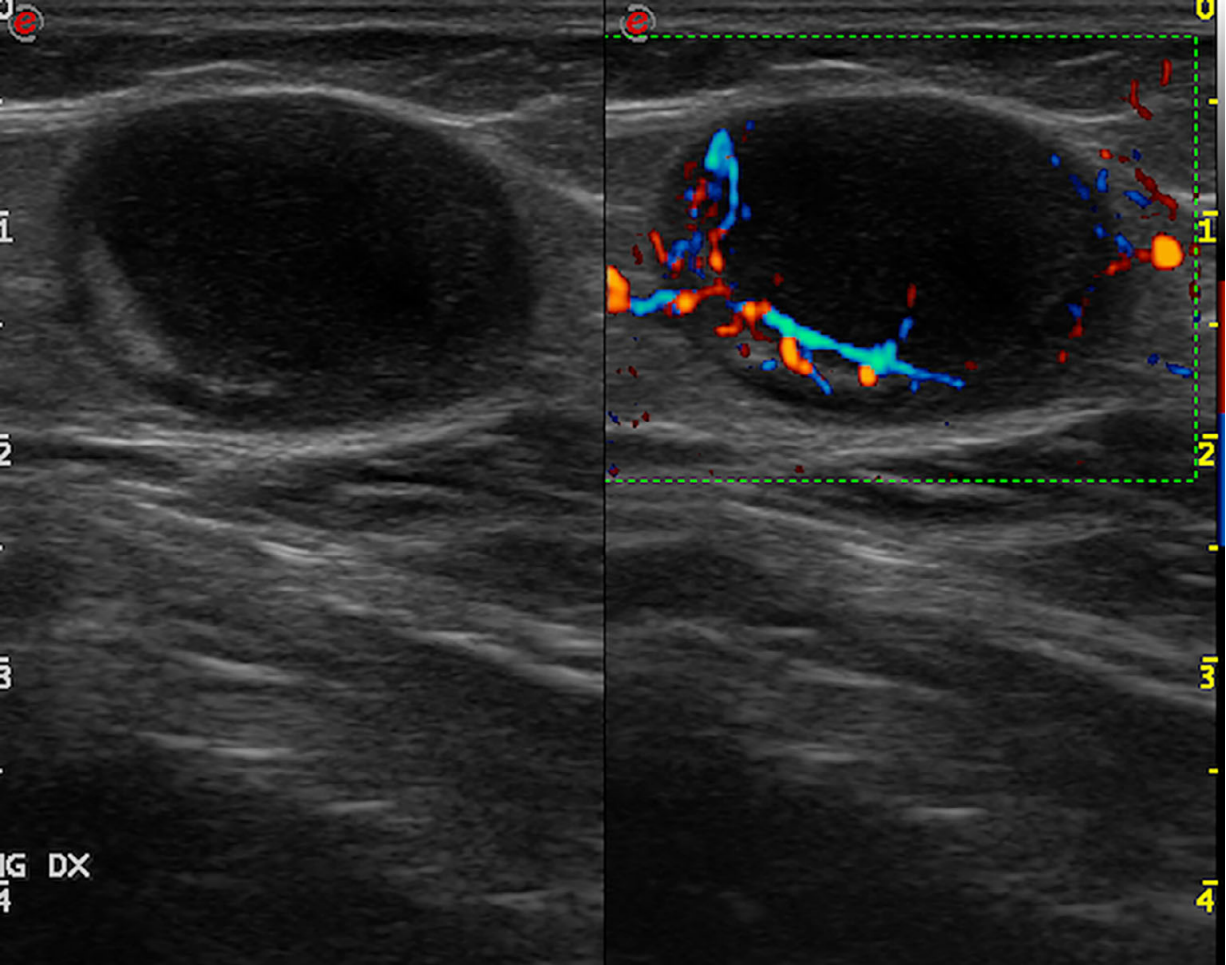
Regional lymph‐node melanoma metastasis to the groin. B‐mode (left) and directional power‐Doppler imaging scan (right). Large, hypoechoic tumor deposit displacing the anatomic and the vascular hilum.
